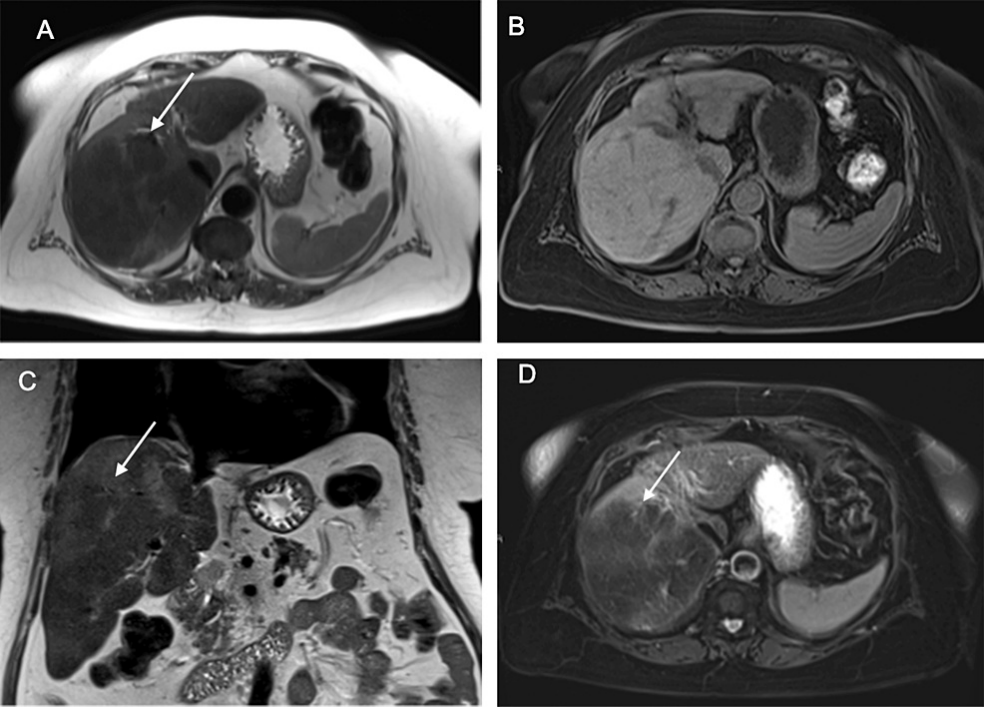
Contrast-enhanced MRI liver (Primovist) (June 2022) The mass appears mildly T2 hypointense relative to the liver parenchyma on the T2-weighted image (A) and T2 fat-saturated image (D). It appears isointense on the T1-weighted image (B). It is difficult to appreciate on the coronal T2-weighted image (C) MRI: magnetic resonance imaging
